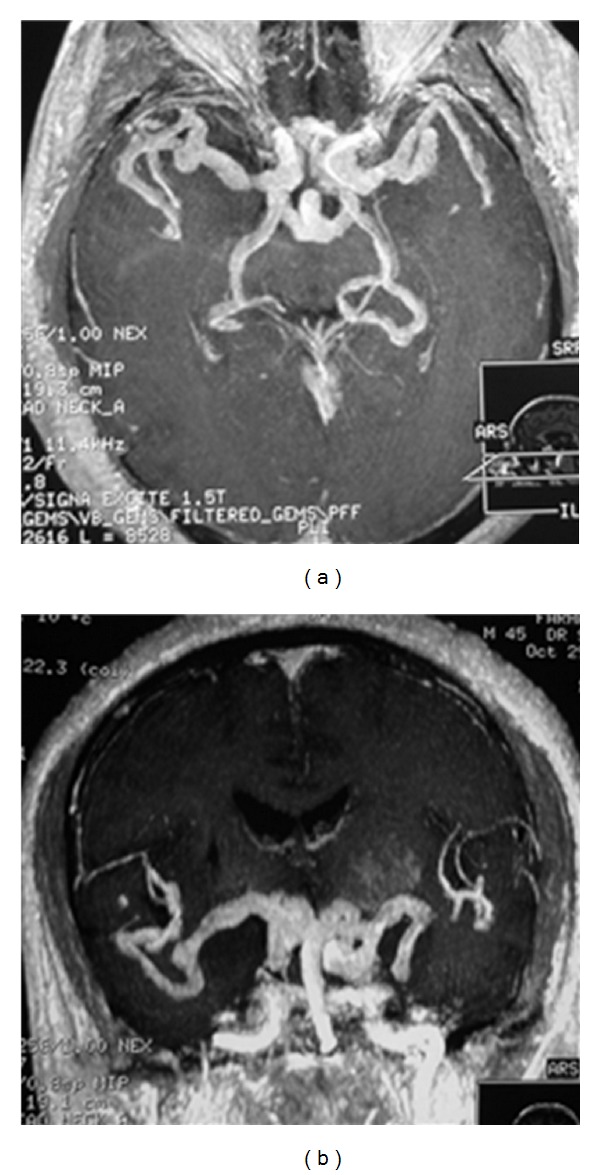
Axial and coronal cerebral MRI T1 gadolinium demonstrated several aneurysms of cerebral arteries.
